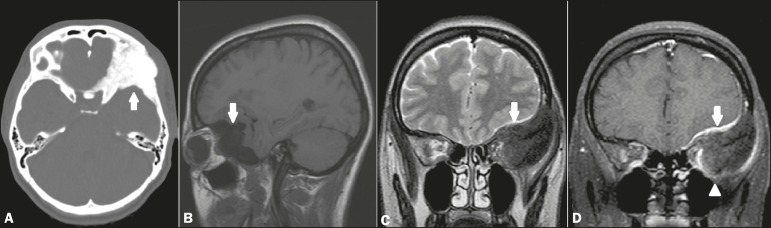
A: Axial CT scan, with bone window settings, showing an
